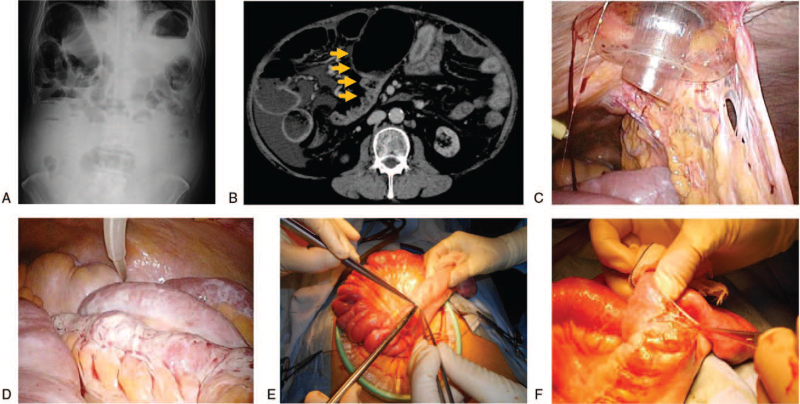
Imaging studies and surgical findings. (A) Plain abdominal radiography showing dilated bowel loops with air-fluid levels. (B) Contrast-enhanced computed tomography scan showing dilated loops of small bowel proximal to the site of obstruction near the ileocecum (arrowheads), with no peritoneal calcification or thickening. (C) The peritoneal dialysis catheter is surrounded by intraperitoneal adhesions. (D) Laparoscopy showing a whitish membranous material wrapped around the bowel. (E and F) A stricture of the terminal ileum is visible, covered with sclerotic peritoneum, which was carefully decorticated.
